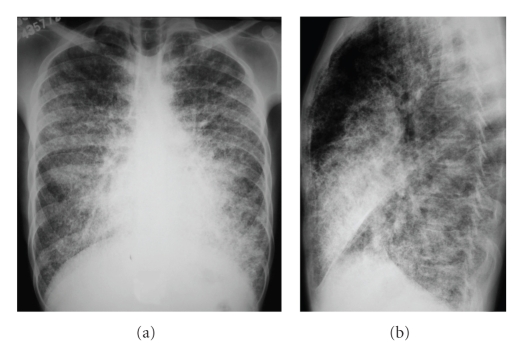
Chest radiographs in anteroposterior (a) and lateral (b) incidences shows a diffuse symmetric lung lesion with confluence of dense micronodular infiltrate. Note the, predominance of the lesions in the paracardiac regions of the lungs.
